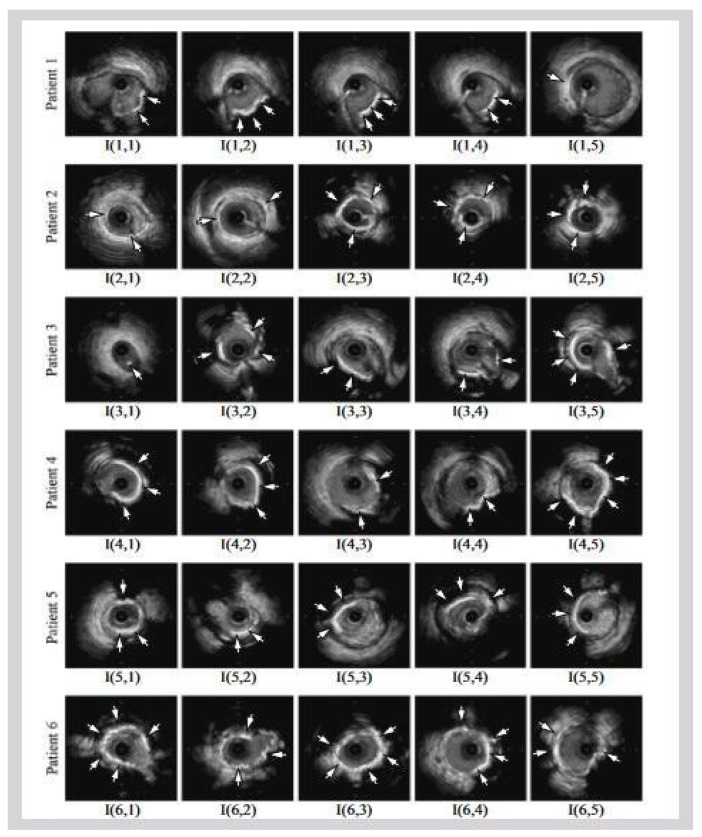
Six patients’ calcified plaques can be seen in sample frames I (1,1) through I (6,5) from the overall intravascular ultrasonography (IVUS) films [43].
